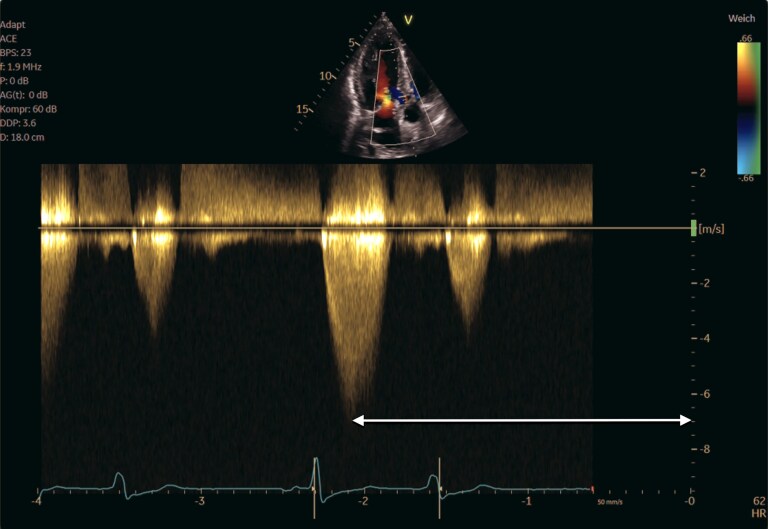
Continuous-wave Doppler assessment across the left ventricular outflow tract. The Doppler spectrum demonstrates a markedly elevated peak velocity (Vmax 7.3 m/s), corresponding to a resting peak gradient of 212 mmHg and a mean gradient of 105 mmHg. These findings indicate severe subvalvular obstruction due to a rigid, hypertrophied muscular ridge within the LVOT.
